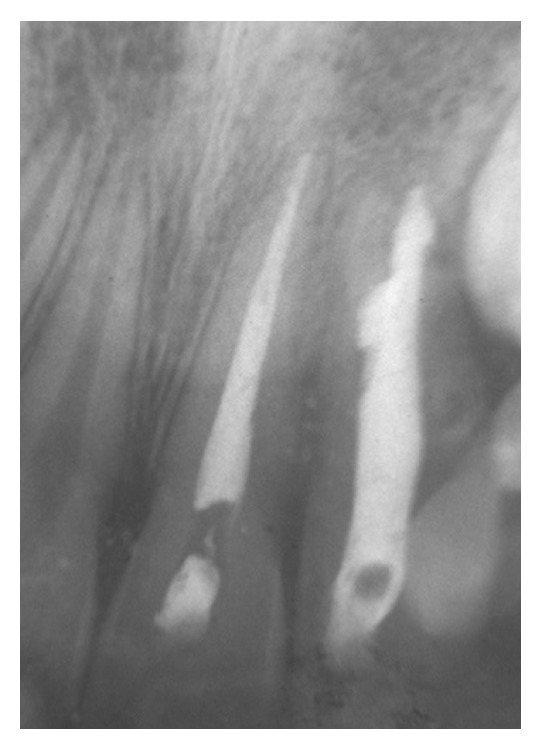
Periapical radiograph showing the root canal treatment of tooth 21 after 1 month (third session; April 1998).
